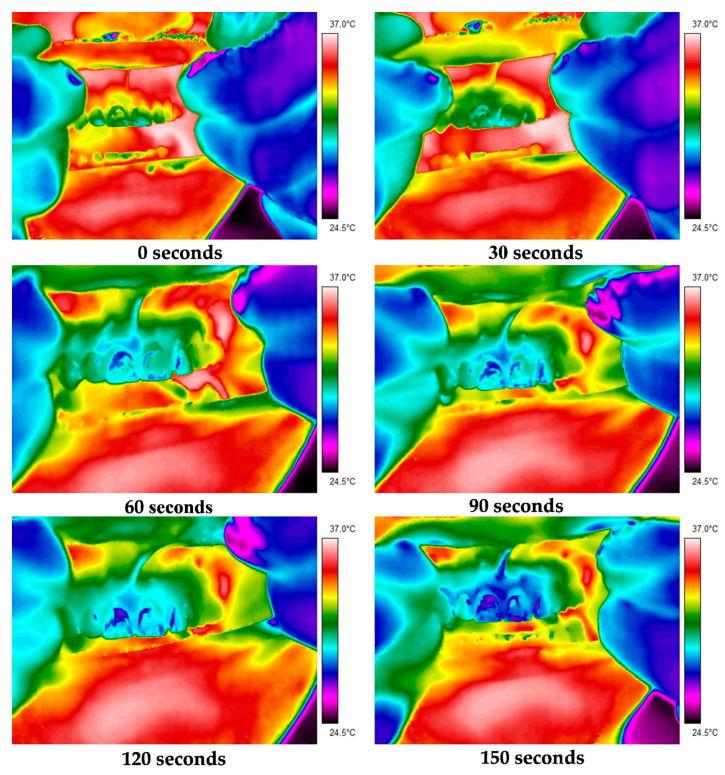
Thermal images of patient I: teeth 21 and 22 after endodontic treatment without periapical lesions; contralateral healthy teeth 11 and 12.
